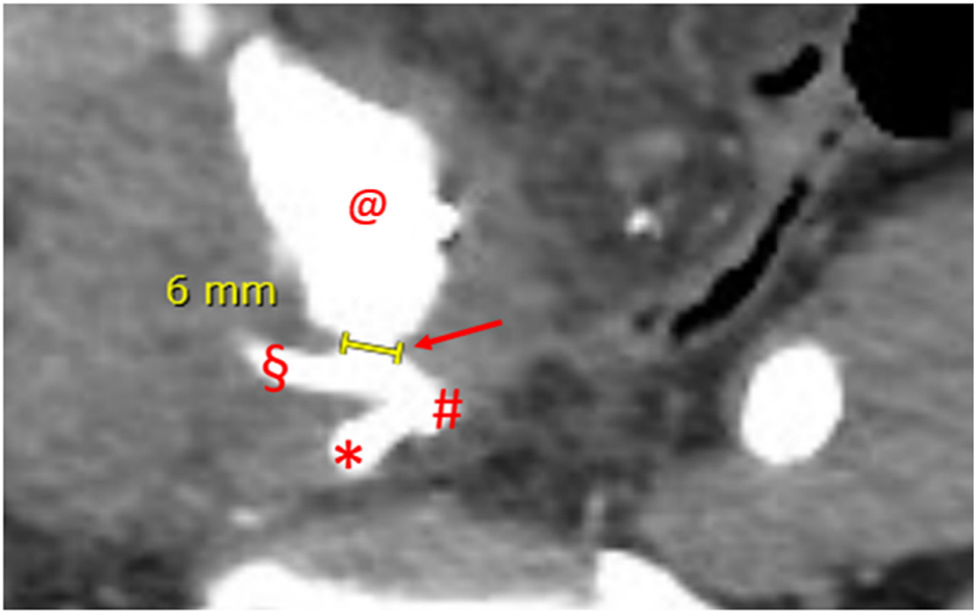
Angio-CT, axial plane, arterial phase, case 1. Major diameter of the UAF. Dart: UAF with concomitant iodinated contrast leakage; #: common right iliac artery bifurcation; *: hypogastric artery; §: external iliac artery; and @: pseudoaneurysm sac.
